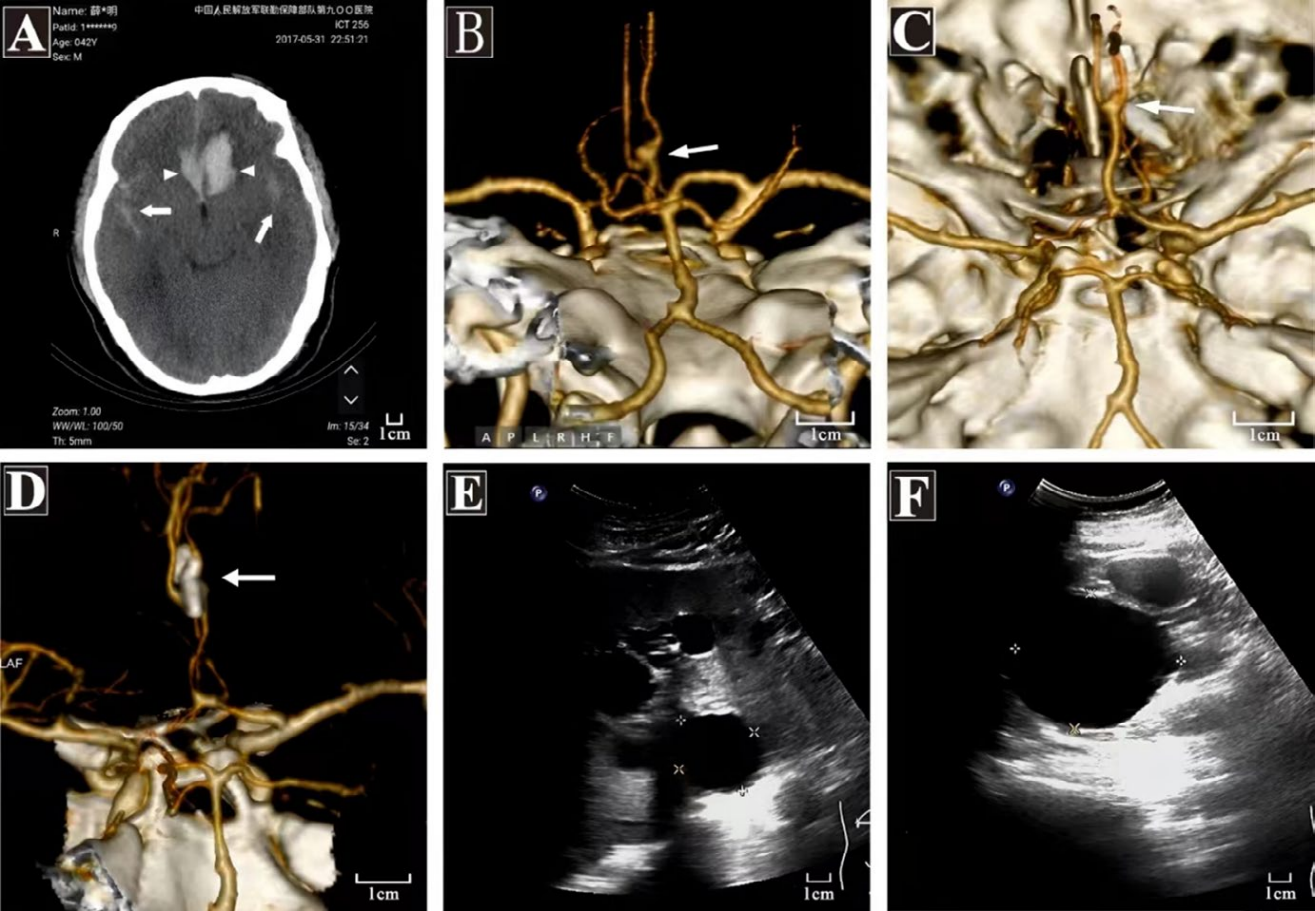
Head computed tomography (CT), CT Angiography (CTA), and abdomen color Doppler ultrasound of proband II:7. (A) Head CT scan showing Fisher grade III subarachnoid hemorrhage with a prefrontal lobe cerebral hematoma (white short arrow). The bleeding area is 3.2 × 2.8 cm, and it is located in the anterior interhemispheric cistern, interpeduncular cistern and bilateral sylvian cistern. High‐density shadow filling (long white arrow) was observed in all cases. (B and C) Preoperative CTA showed a fusiform aneurysm (white arrow) in the A2 segment of the left anterior cerebral artery, approximately 1.1 × 0.8 mm in size. (D) Postoperative review CTA demonstrating correct placement of the titanium clip at the aneurysm site. (E) Color Doppler ultrasound of the left kidney cyst. (F) Color Doppler ultrasound liver cyst.
